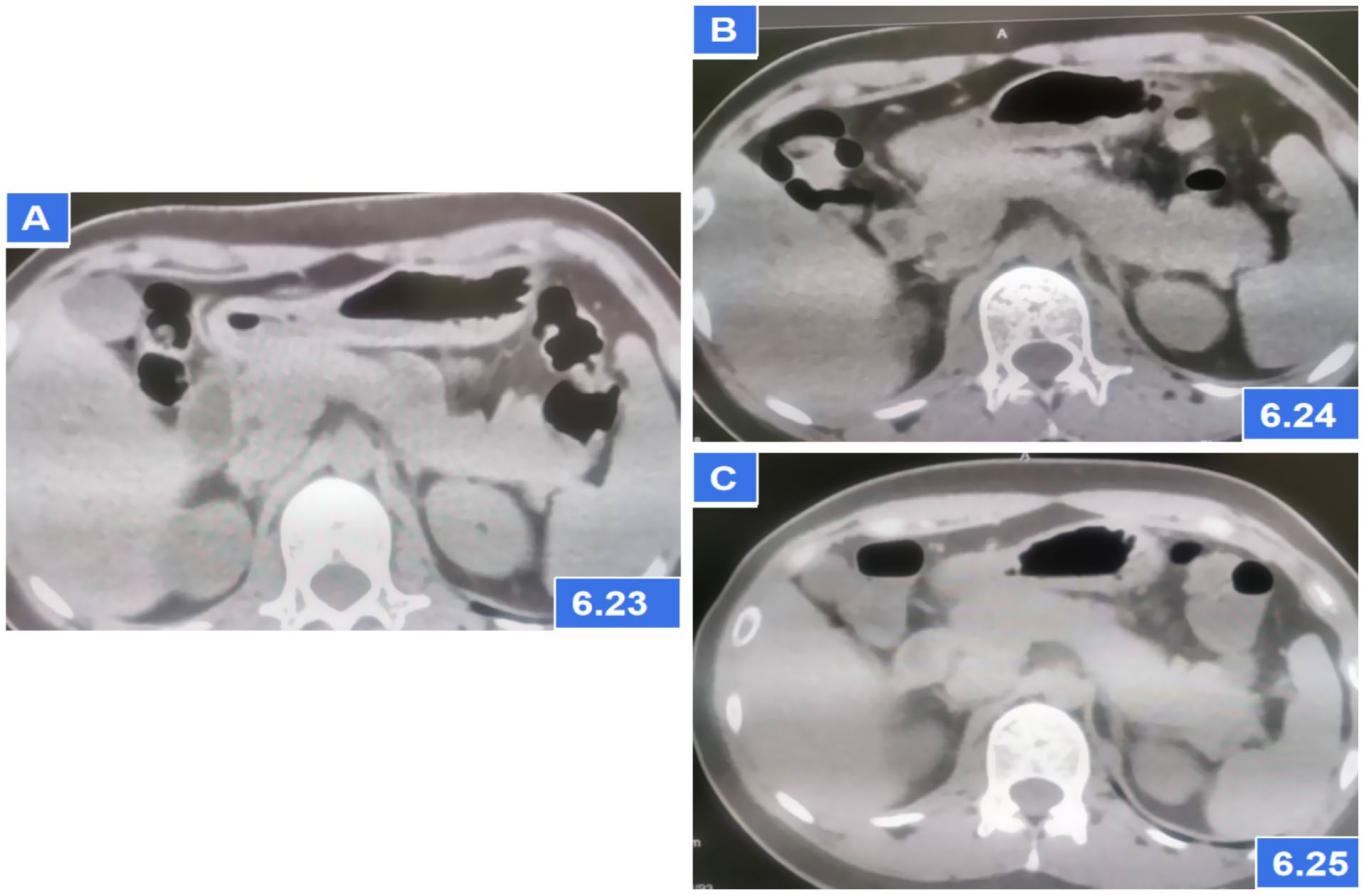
Abdominal CT showing pancreatic parenchymal swelling; (A) CT scans on the first day after admission; (B) CT scans at 6 h later after admission; (C) CT scans after AP diagnosis and treatment for 2 days.
